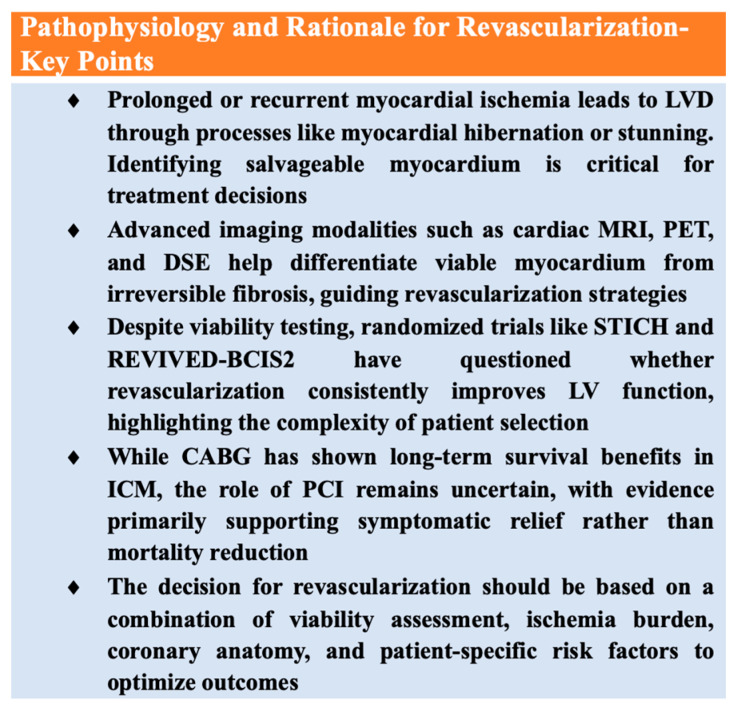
Pathophysiology and rationale for revascularization in ischemic cardiomyopathy. DSE, dobutamin stress echocardiography; ICM, ischemic cardiomyopathy; LV, left ventricular; LVD, left ventricular dysfunction; MRI, magnetic resonance imaging; PCI, percutaneous coronary intervention; PET, positron emission tomography; REVIVED-BCIS2, Study of Efficacy and Safety of Percutaneous Coronary Intervention to Improve Survival in Heart Failure; STICH, Surgical Treatment for Ischemic Heart Failure.
